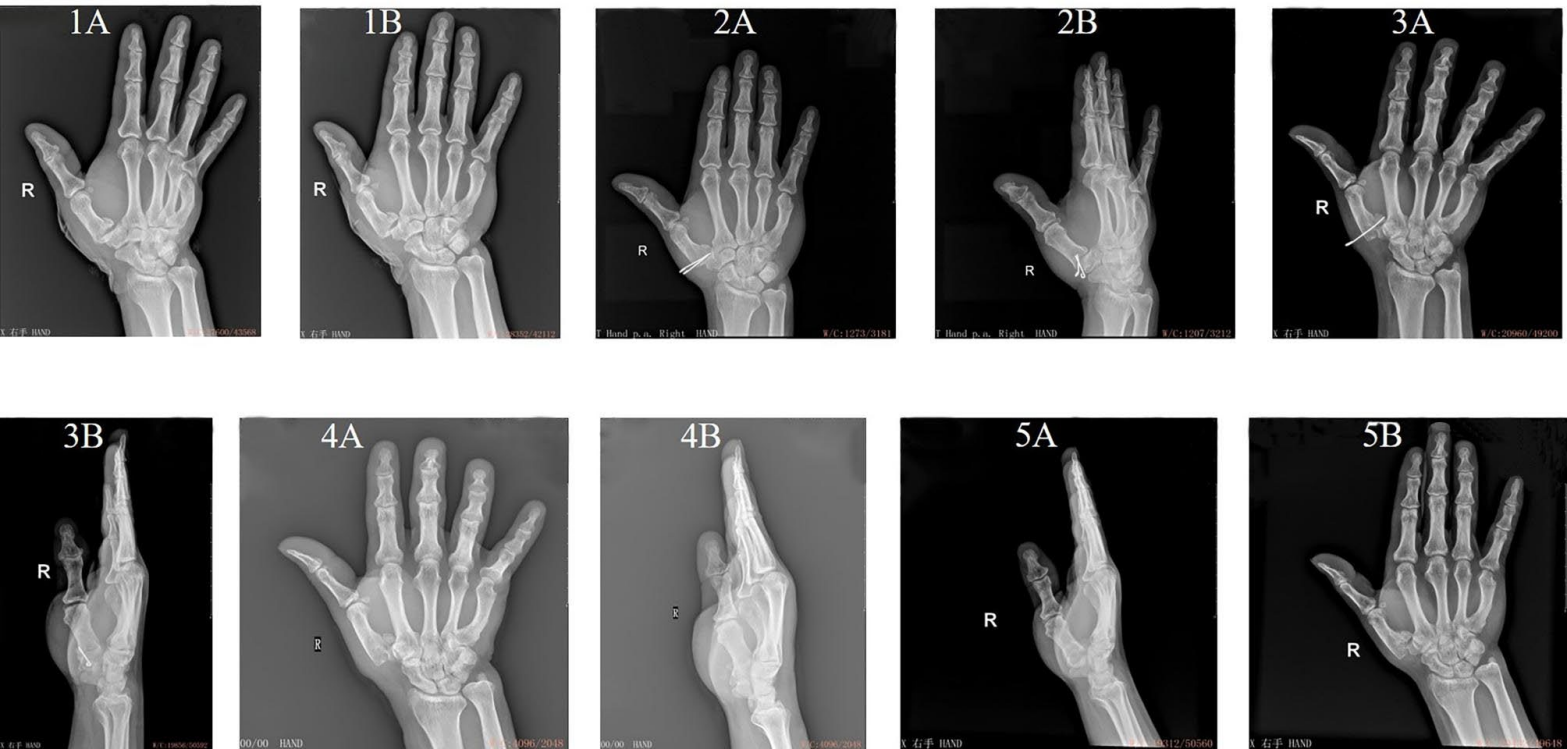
Representative clinical treatment course of a patients treated with external fixation combined with Kirschner wire fixation and suffered complications after operation. (1 A and 1B) X-ray examination before operation showed comminuted base fracture of first metacarpal bone. (2 A and 2B) X-ray examination one month after operation showed that the first carpometacarpal joint surface was restored, and the first carpometacarpal joint was in position. (3 A and 3B) X-ray examination showed that the loosening of external fixation without timely adjustment resulted in fracture displacement and internal fixation failure. (4 A-B) X-ray examination showed that the Kirschner wires were removed. (5 A-B) X-ray examination showed that the first carpometacarpal joint surface was restored, and the first carpometacarpal joint was in position finally
